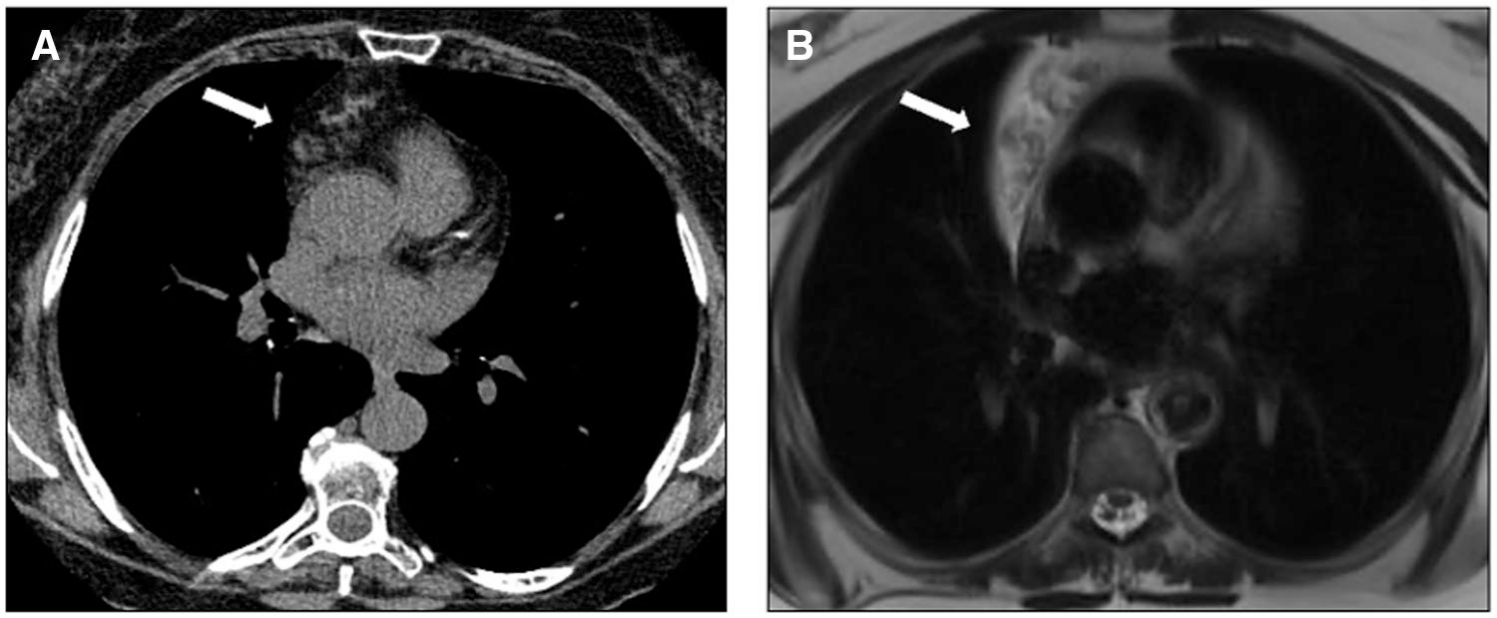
Thymolipoma. (A) Non-contrast axial CT image shows a fatty mass in the right prevascular compartment intermixed with linear whorls of soft-tissue (arrow), corresponding to adipose and thymic tissue, respectively. (B) Axial T2-weighted MR image of the chest shows the prevascular mass (arrow), characterized by predominant high-signal intensity due to the fat component and intermediate signal intensity from the thymic tissue.
